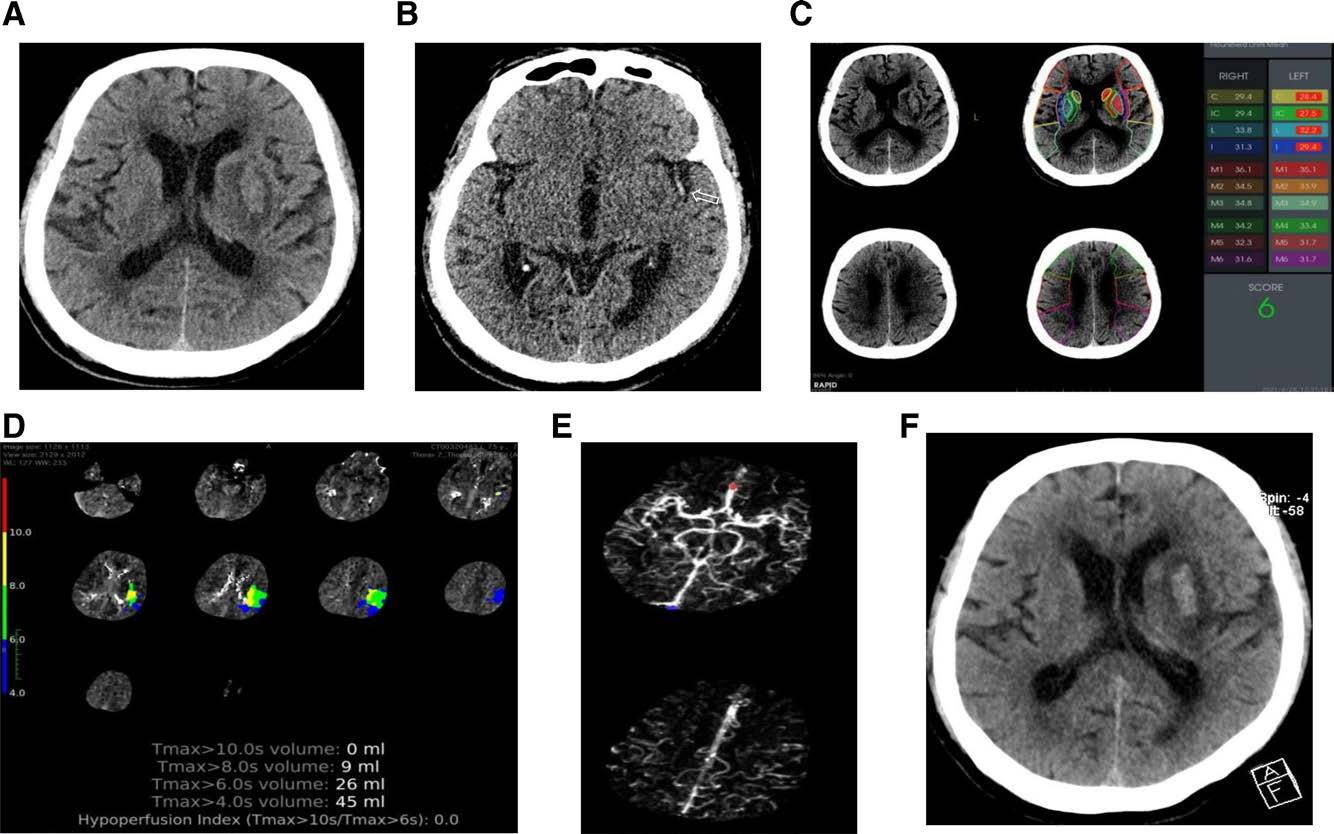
CT images of a patient with infarct in the left cerebral hemisphere. (A) Hypoattenuation in the left basal ganglia and isodensity in the central area (lenticular nucleus) of the hypoattenuation region at the 10th hour of onset. (B) Hyperdensity sign (white arrow) at the MCA M2 segment. (C) RAPID CT scans showed the infarct in the lenticular nucleus, caudate nucleus, internal capsule, and insula, and the RAPID score was 6. (D) Hypoperfusion in the territory of the upper trunk of the left MCA on perfusion CT scans. (E) CT angiography demonstrated the left MCA M1 segment and slightly fewer distal branches than the right. (F) After 3 days of onset, the hypoattenuation region’s center area (lenticular nucleus) changed from isodensity to slightly higher density on CT scans. CT = computed tomography, MCA = middle cerebral artery.
